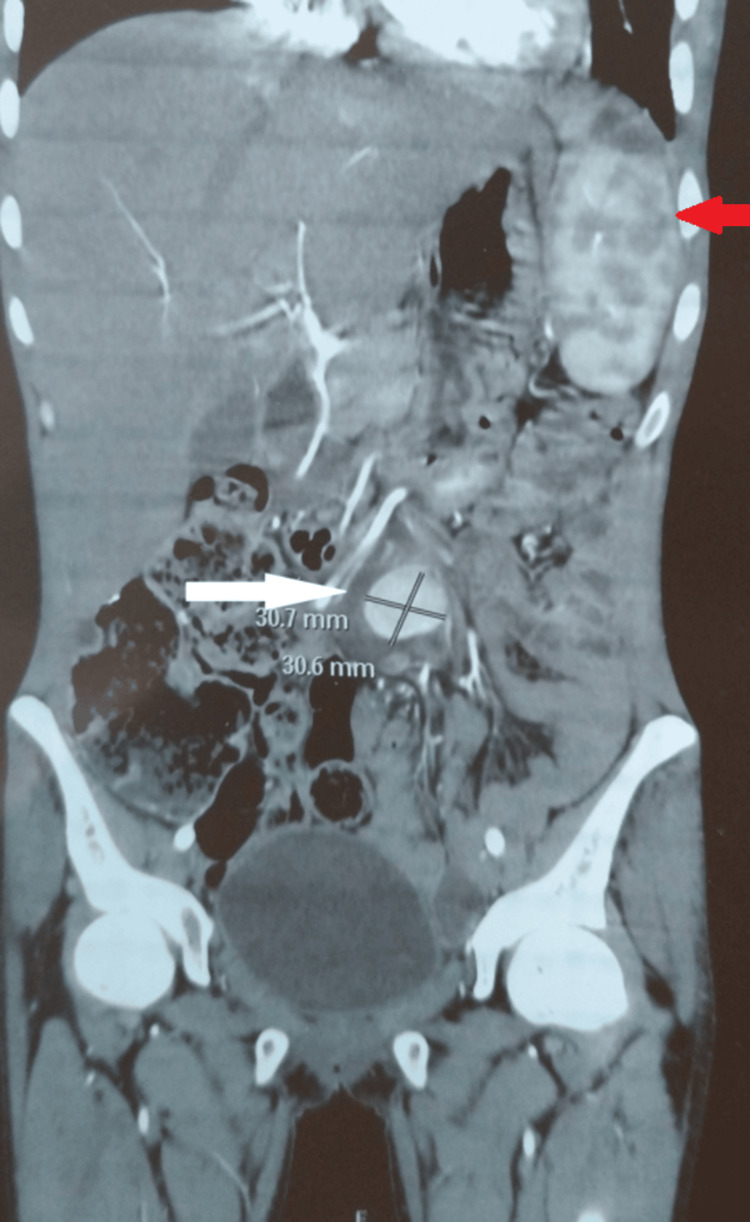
An abdominal CT scan with intravenous contrast (coronal view) shows abnormal aneurysmal dilation of the superior mesenteric artery filled with contrast (white arrow), along with multiple splenic infarctions (red arrow).
